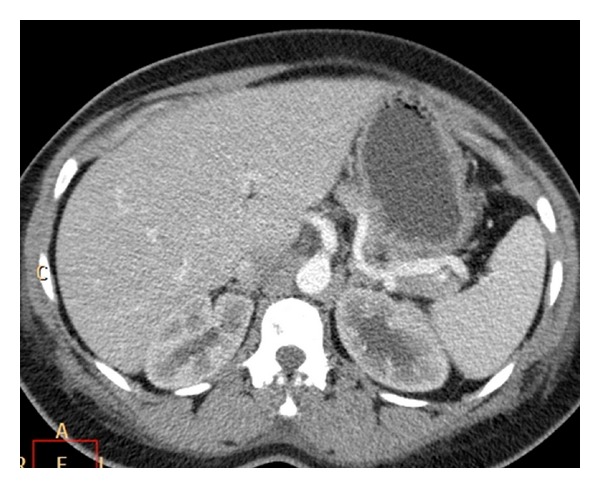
Follow-up CT abdomen after a week shows resolution of gastric emphysema.
